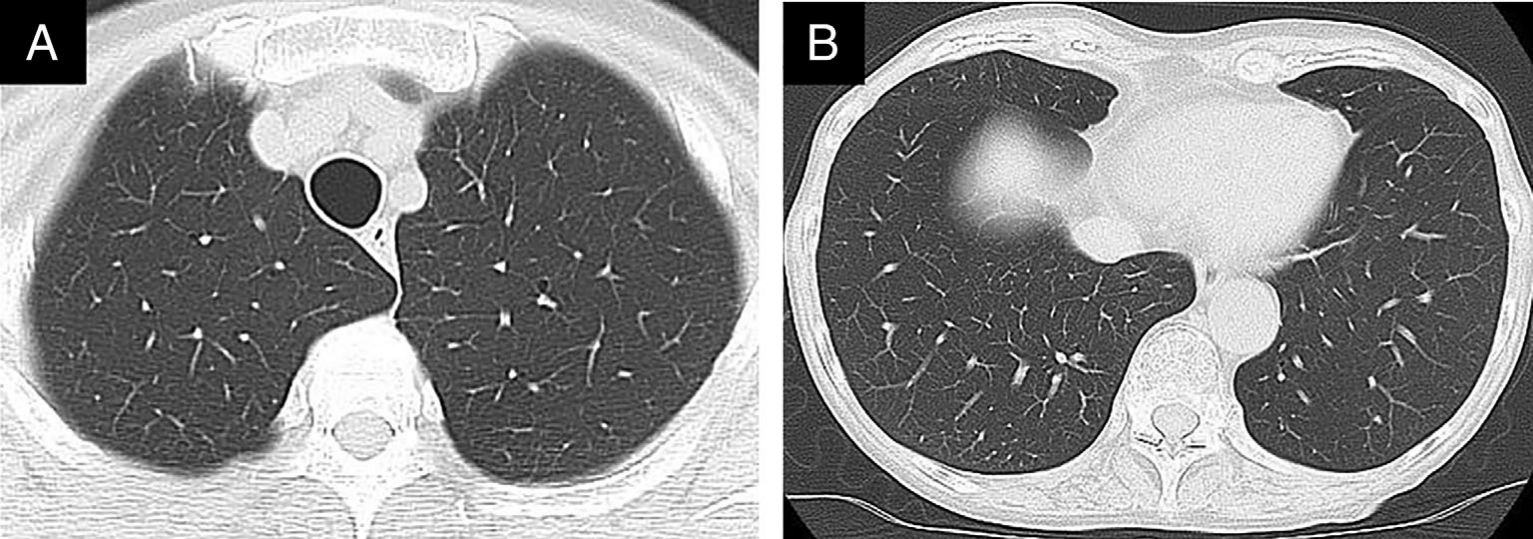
Chest computed tomography (CT) findings two weeks after corticosteroid treatment. Chest CT obtained two weeks after corticosteroid treatment showed substantial improvement in the interstitial shadows both in bilateral upper (A) and lower (B) lobes.
